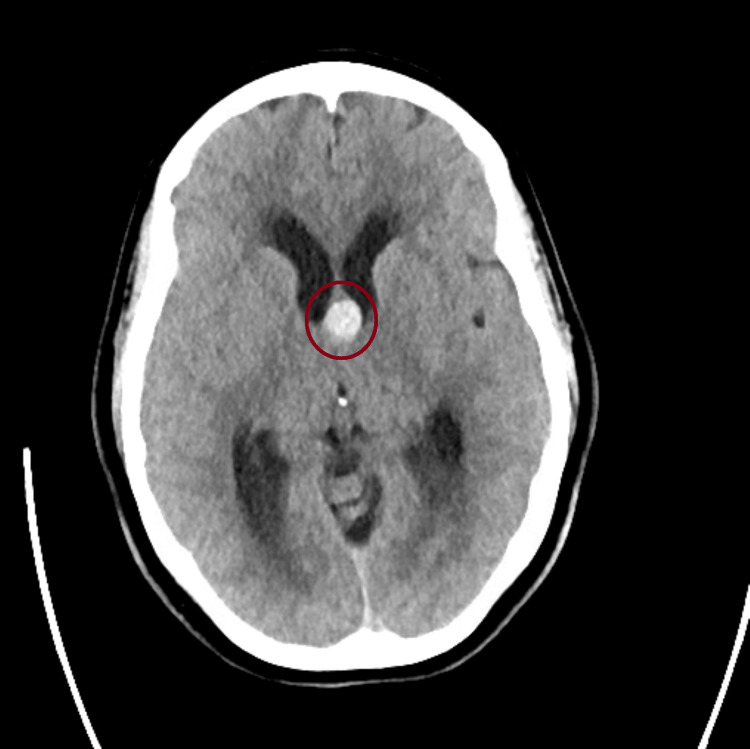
Coronal non-contrast CT showing a hyperdense colloid cyst at the foramen of Monro with associated ventricular enlargement.
